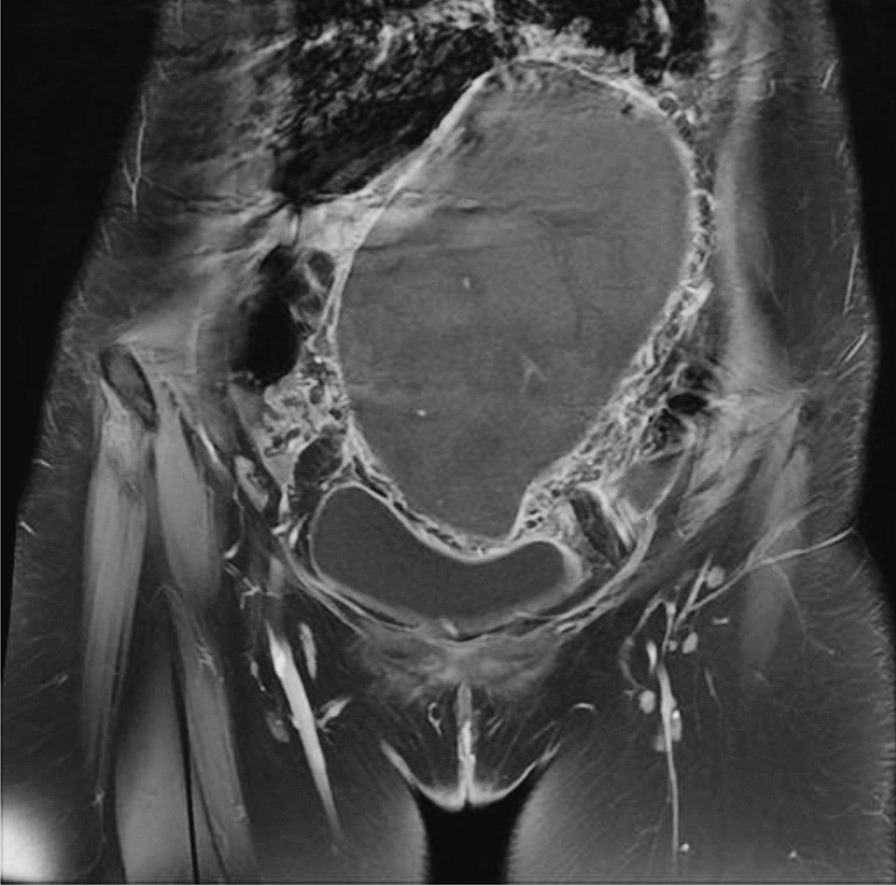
MRI pelvis showing a large obliquely placed mass. Swirling of the vessels can be noted on the left side
